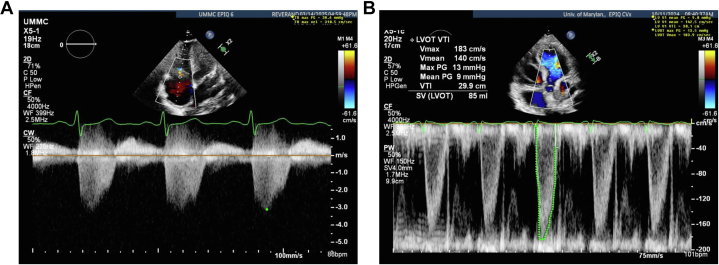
Transthoracic Echocardiography Prior to Pregnancy First presentation to the emergency department for dyspnea on exertion, immediately before pregnancy. (A) Tricuspid valve regurgitation with a TR max velocity of 385.0 cm/s, correlating with a right ventricular systolic pressure of 67.3 mm Hg. (B) The LVOT VTI is 29.5 cm, significantly elevated, consistent with volume overload. LVOT = left ventricular outflow tract; PG = pressure gradient; SV = stroke volume; TR max velocity = tricuspid regurgitation maximum velocity; Vmax = maximal velocity; Vmean = mean velocity; VTI = velocity time interval.
